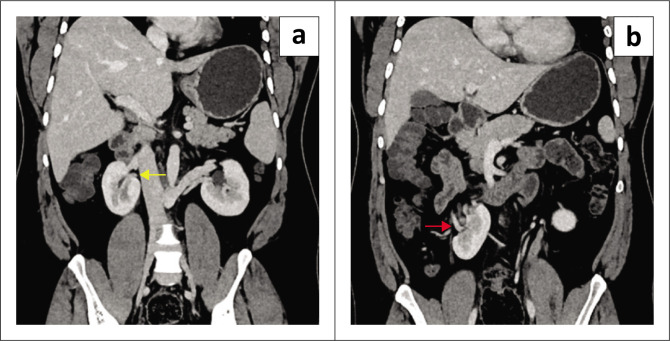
Venous phase contrast-enhanced CT. (a) Coronal reconstruction demonstrating the pelvicalyceal system of the cranially located right kidney facing medially (yellow arrow). (b) Coronal reconstruction with the pelvicalyceal system of the caudally located right kidney facing laterally (red arrow).
